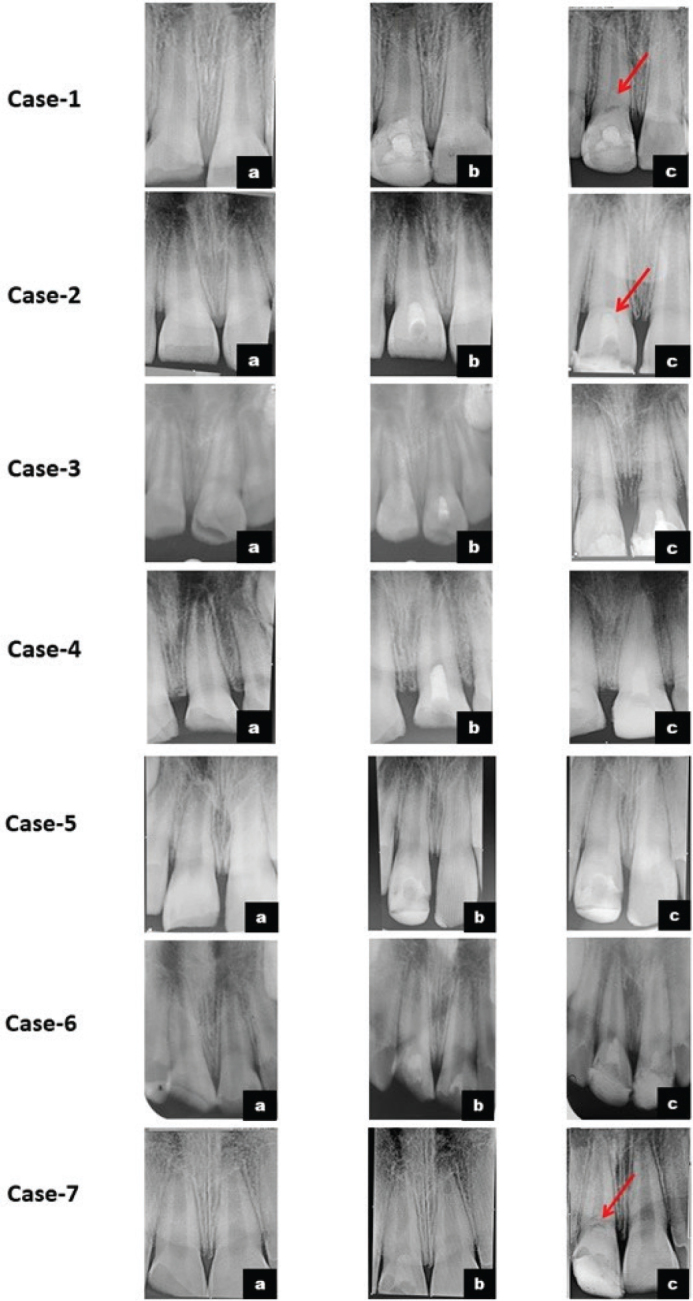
Intra-oral periapical radiographs of cases 1–7. a- pre-operative; b- immediate post-operative; c- post-operative at last follow-ups with evidence of continued apexogenesis; red arrow marks dentin bridge formation.
